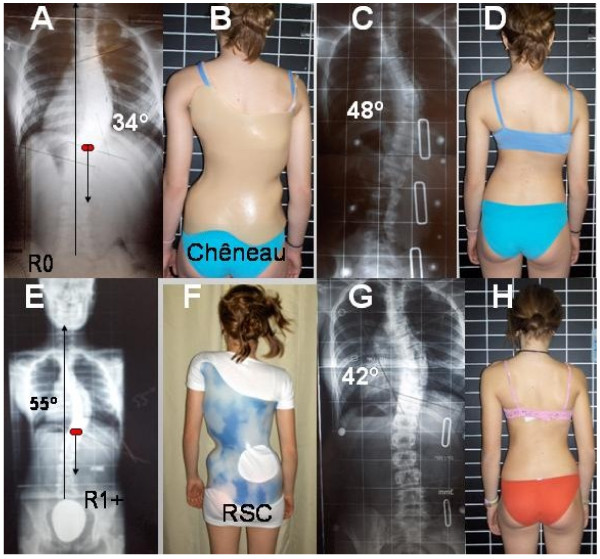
Wrong and right brace design according to curve pattern. A 11-year old girl with scoliosis of 34° Cobb (A), treated with a four-curve scoliosis brace (B), progressing to 48° (C) in brace and 55° (E) out of brace after one year of treatment (D). A brace well designed according to her classification of a three curves- A2 type (F) produced an acceptable in brace correction (G) with a good clinical response (H). She had criteria to be diagnosed as a three curves pattern from the first X-Ray.
